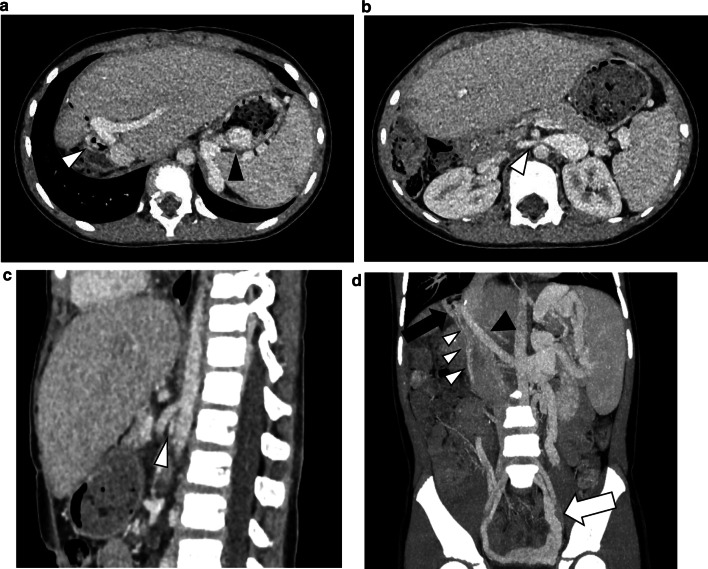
AB Axial CT image. a The white arrowhead shows the jejunal varices. The black arrowhead shows the gastric varices. b The white arrowhead indicates compression of the LRV between the SMA and the Ao. c Sagittal CT image. The white arrowhead indicates the narrow angle between the SMA and the Ao. d Multiplaner reconstruction CT image. The black arrow shows the varices of the RY jejunum. The white arrowheads indicate collaterals to the RY varices from the SMV. The black arrowhead indicates the patent RPA. Pelvic vein congestion (white arrow) is suggestive of increased venous pressure via the dilated gonadal vein. *Ao* abdominal aorta, *CT* computed tomography, *LRV* left renal vein, *RY* Roux-en-Y, *SMA* supramesenteric artery, *SMV* supramesenteric vein
